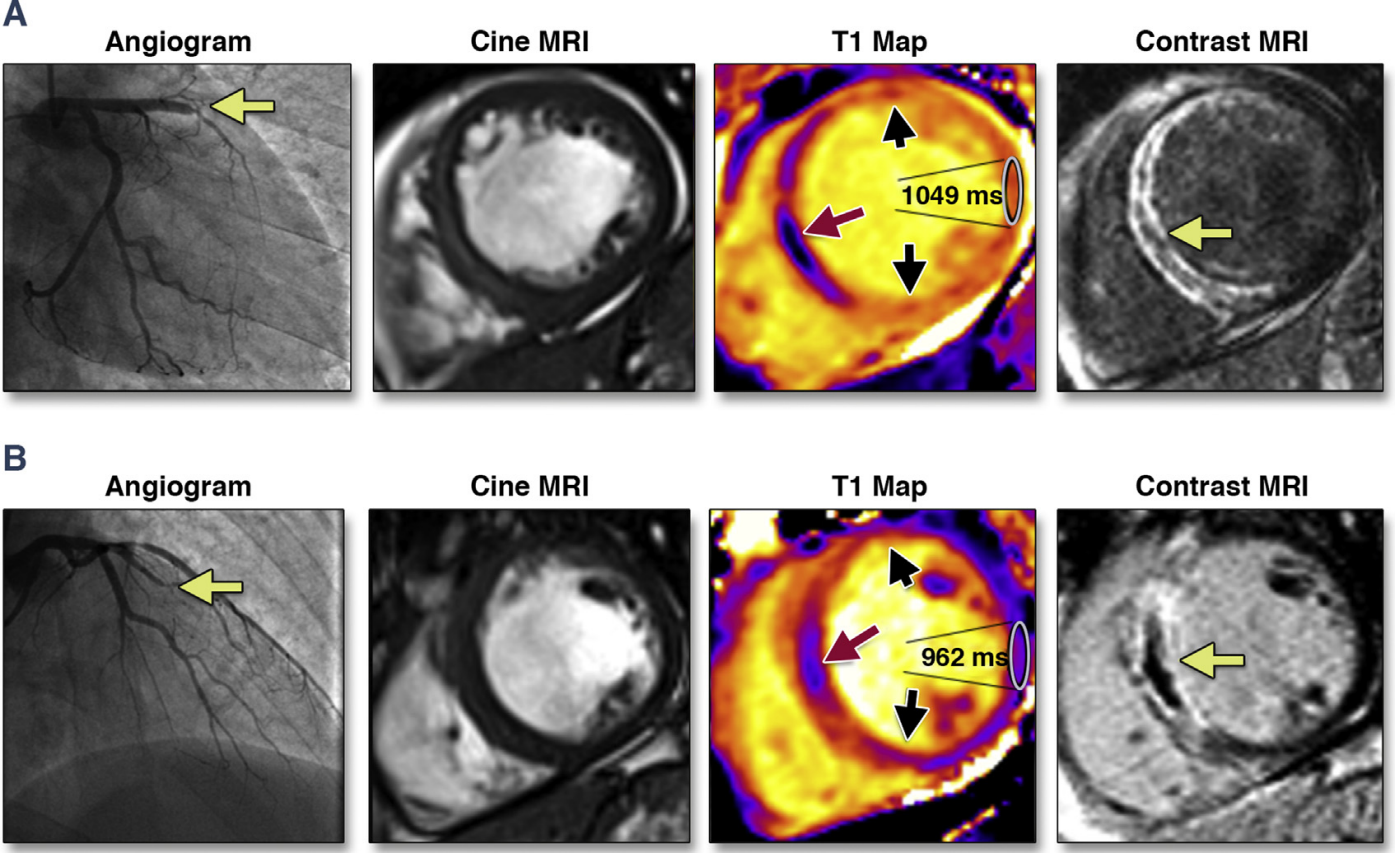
Two Patients With Anterior STEMI and Divergent Clinical Courses Both patients had similar clinical presentations, with acute anterior ST-segment elevation myocardial infarction (STEMI) treated by primary percutaneous coronary intervention. The yellow arrow indicates the location of the thrombotic occlusion of the (A) left anterior descending, and (B) obtuse marginal, branch of the left coronary artery. The history of each patient is described in the Online Appendix. MRI = magnetic resonance imaging.
